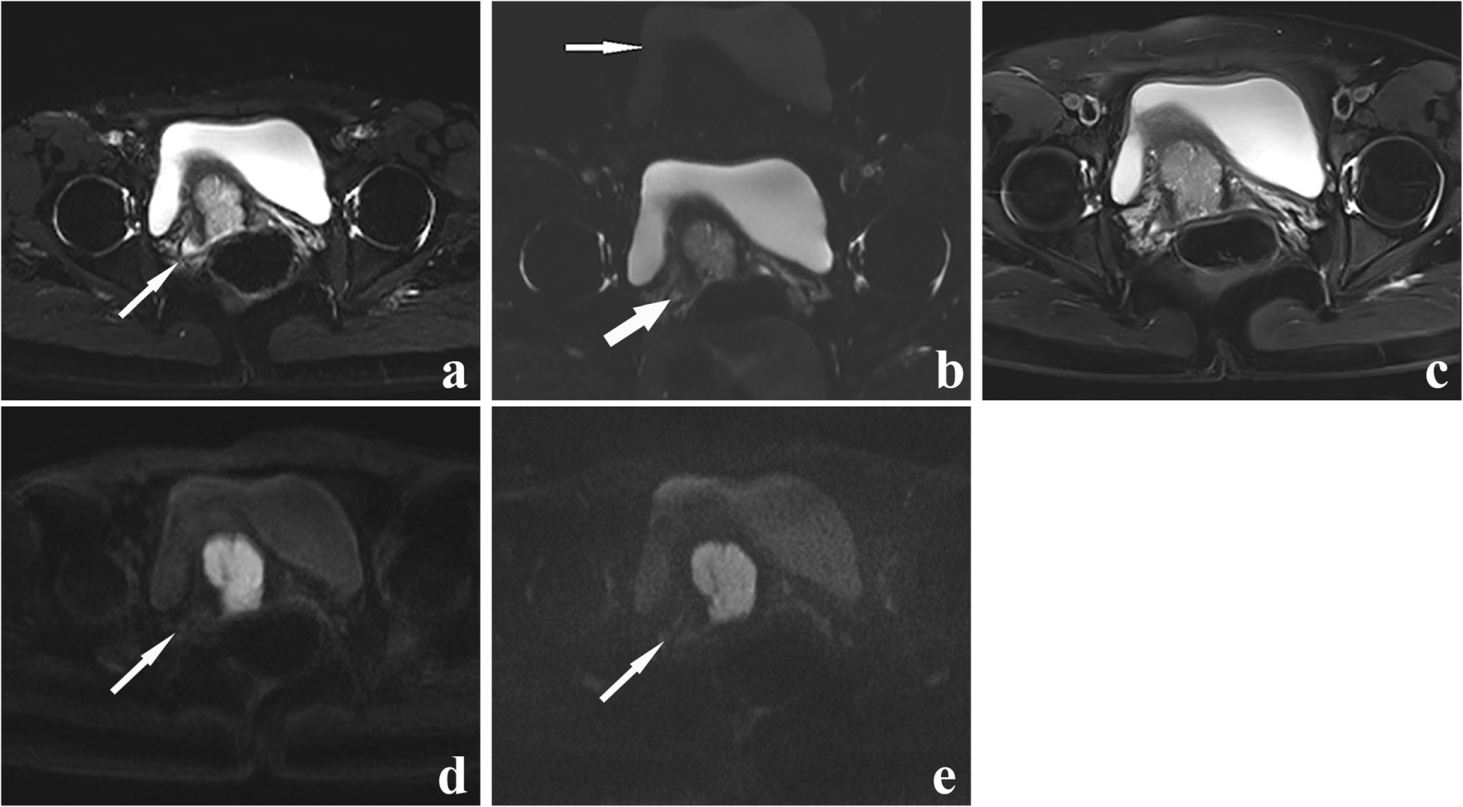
A 54-year-old woman with endometrial cancer (endometrioid carcinoma, moderately differentiated, superficial myometrial invasion). a An axial RESOLVE DWI image (b = 0 s/mm^2^). b an axial SS-EPI DWI image (b = 0 s/mm^2^). c an axial T2WI image. d An axial RESOLVE DWI image (b = 1000s/mm^2^). e an axial SS-EPI DWI image (b = 1000s/mm^2^). The right side of the uterus is presented as a hyperintense signal on (a) (white arrow). (b) showing only a slightly higher signal intensity in the lesion (thick arrow). This suggested endometrial cancer on the right side of the uterus. The artifacts were seen on (b) (thin white arrow). As indicated by the white arrow, the lesion can be seen more clearly in (d) than in (e). Therefore, this case of stage IIIB endometrioid adenocarcinoma was accurately diagnosed by RESOLVE DWI
